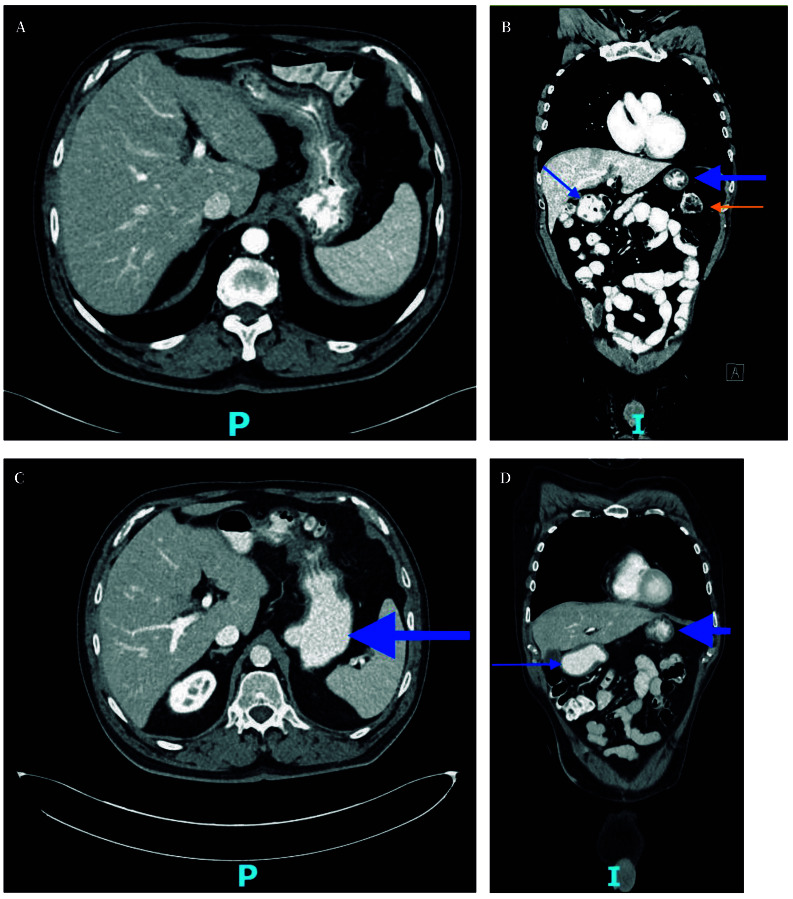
Computed tomography scan. Axial (A) and coronal (B) view at three month follow-up, with almost complete resolution of the gastric bezoar (blue arrow), with some organized-appearing content remaining in the antral region (thin blue arrow). Splenic colon flexure (orange arrow). Axial (C) and coronal (D) view at six month follow-up, with normal transit of oral contrast in gastric chamber (blue arrow) or pyloric antrum (thin blue arrow).
